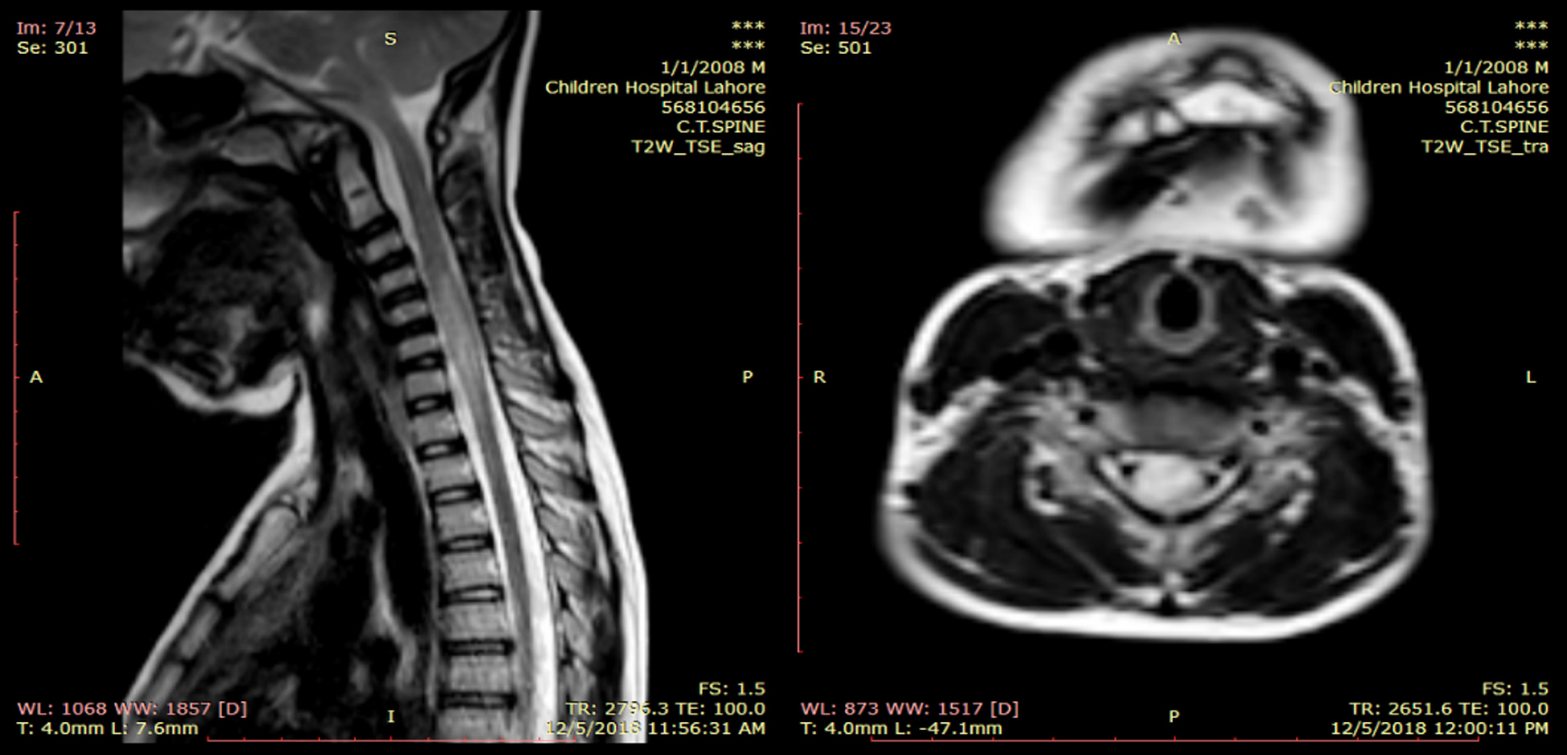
T2 W sagittal and axial MRI through cervical spine. Linear hyperintense area along with swelling within cervical cord (C3-C7) involving upper thoracic cord as well: consistent with long segment Transverse myelitis.
